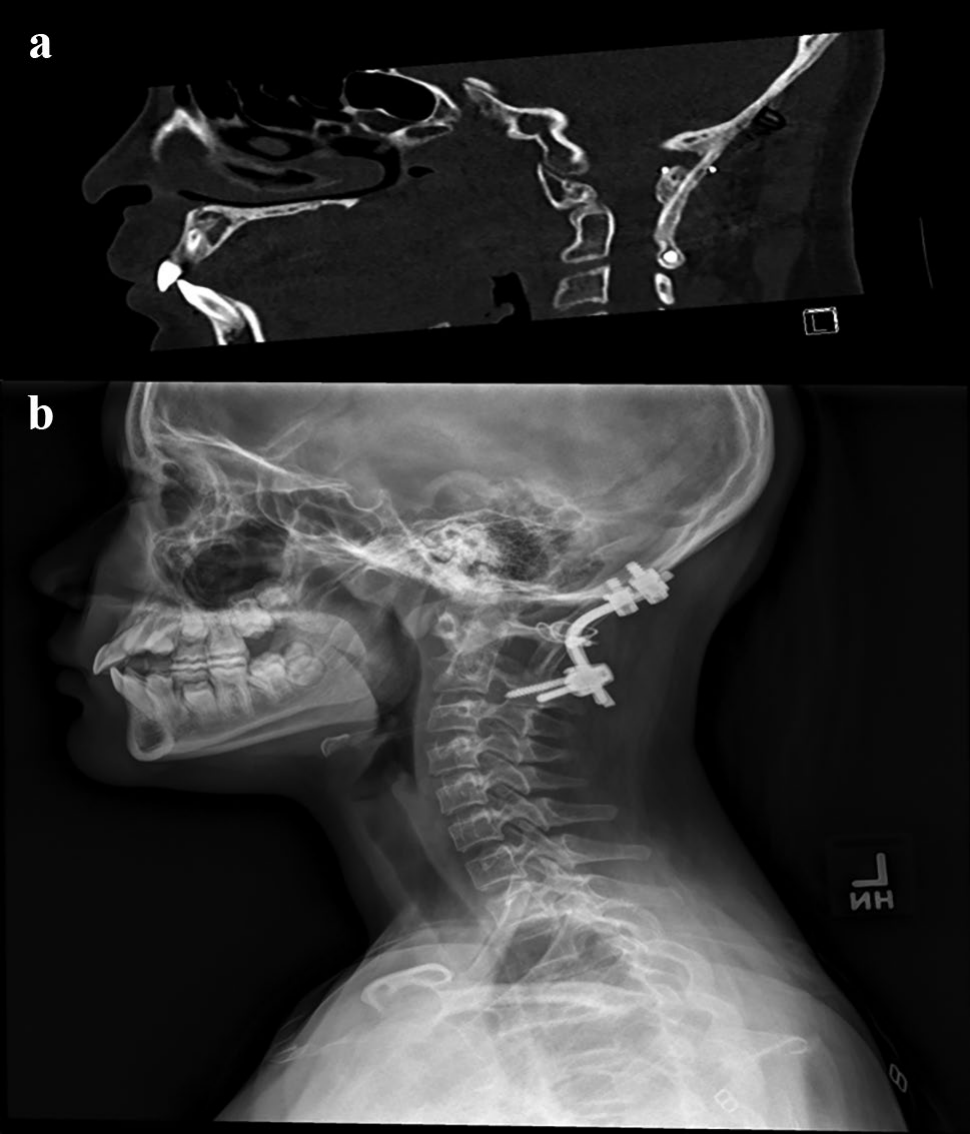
Representative surgical images of fusion construct. a Sagittal CT scan showing presence of rib bone graft between the occiput and C2. b Representation lateral X-ray obtained postoperatively showing implant construct and sublaminar wiring with rib bone graft
